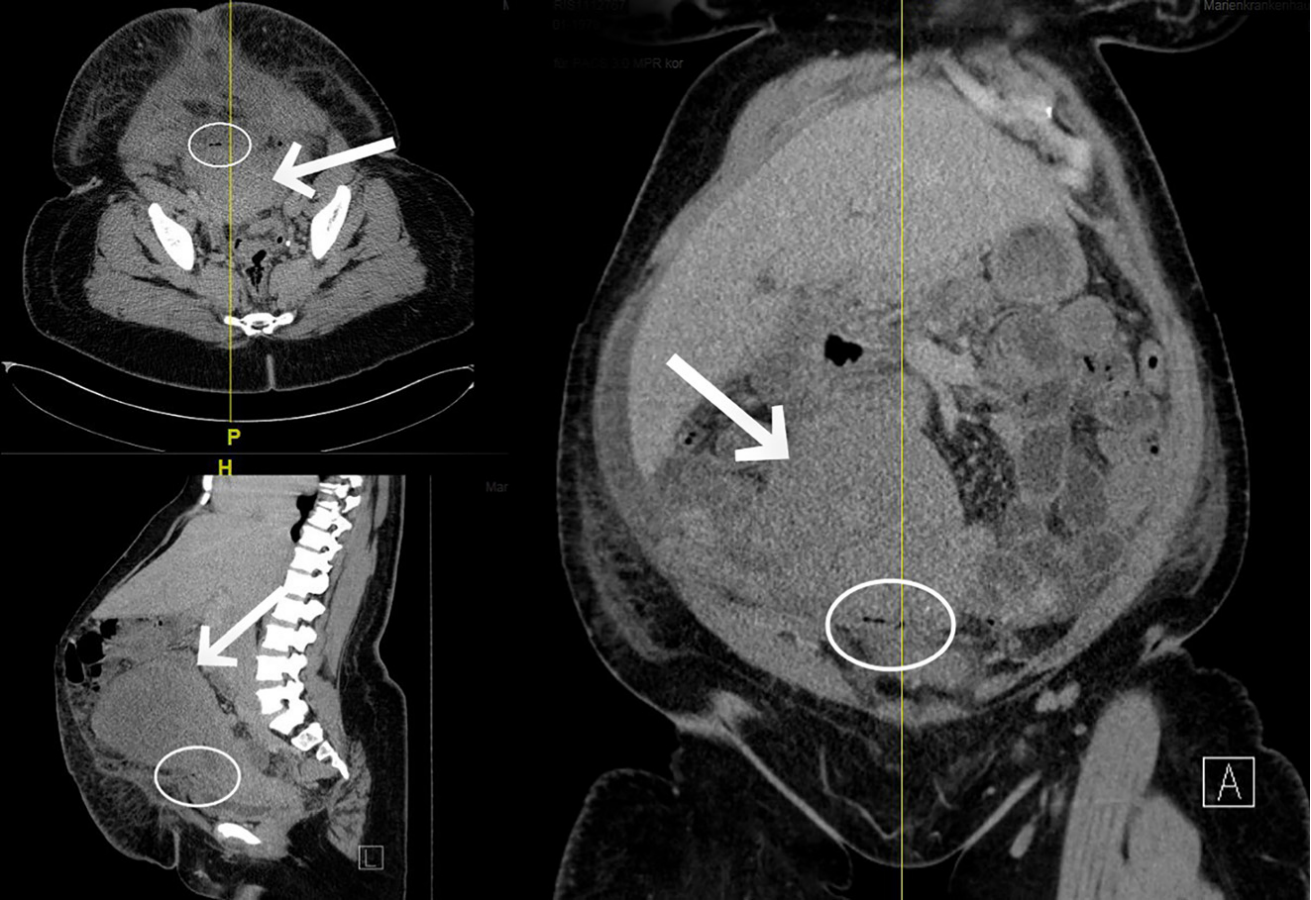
Abdominal CT showing enlarged postpartum uterus (arrows) and few air pockets (circles).
